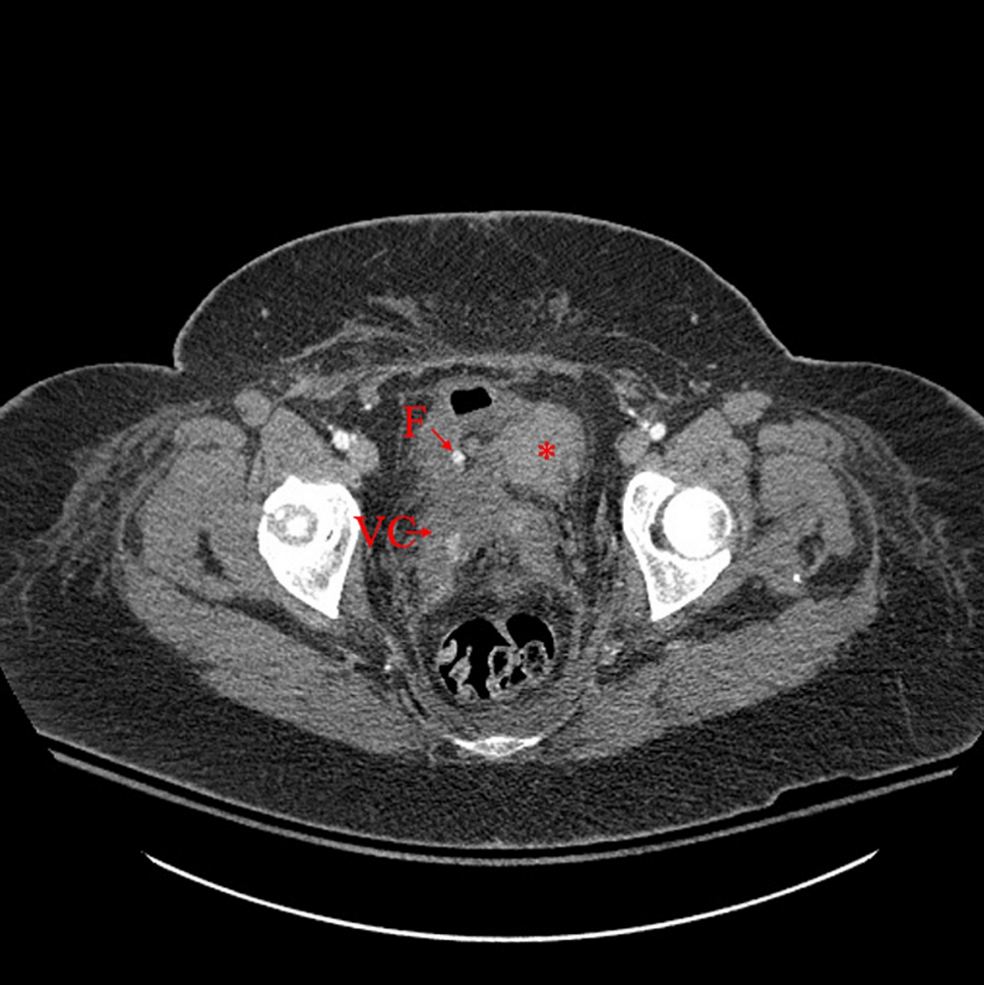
Axial CT demonstrating caudal margin of the retroperitoneal hematoma alongside the vaginal cuff anteriorly, causing mass effect on the bladder to the patient’s midline. VC, vaginal cuff; F, Foley catheter; *, retroperitoneal hematoma
